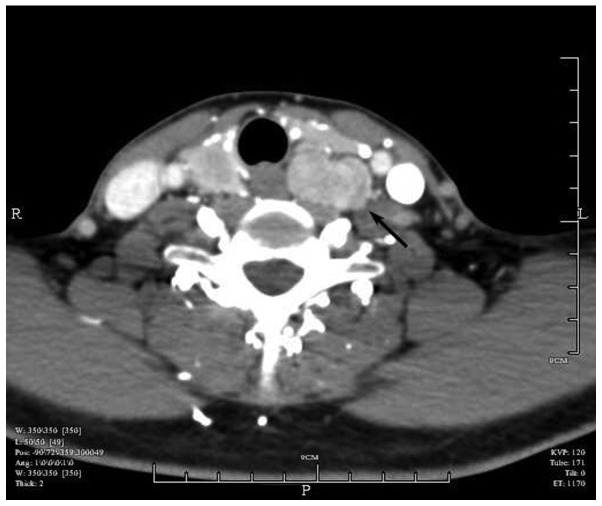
Plain and enhanced computed tomography scan revealed a soft tissue mass in the left posterior side of the thyroid.
